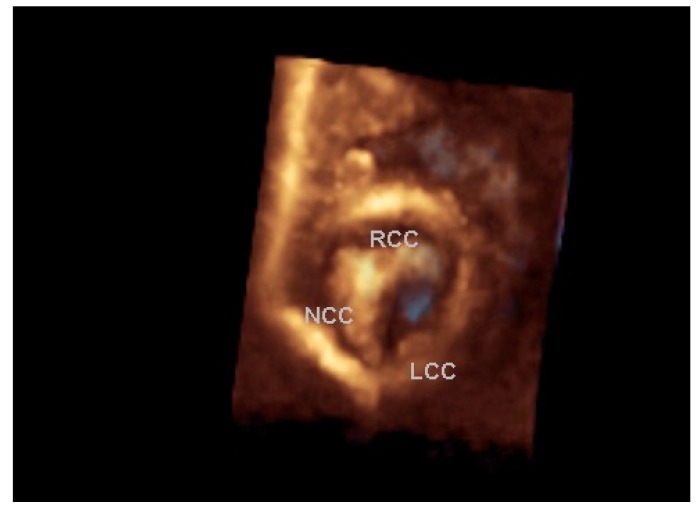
Valvular aortic stenosis with a bicuspid valve. A full volume rendered three-dimensional echocardiographic “surgeon’s view” of a bicuspid aortic valve in systole showing fused right (RCC) and noncoronary (NCC) cusps with prominent ridge, a smaller left coronary cusp (LCC) (lower to the orifice) and an eccentric elliptical restricted orifice (in light blue color).
